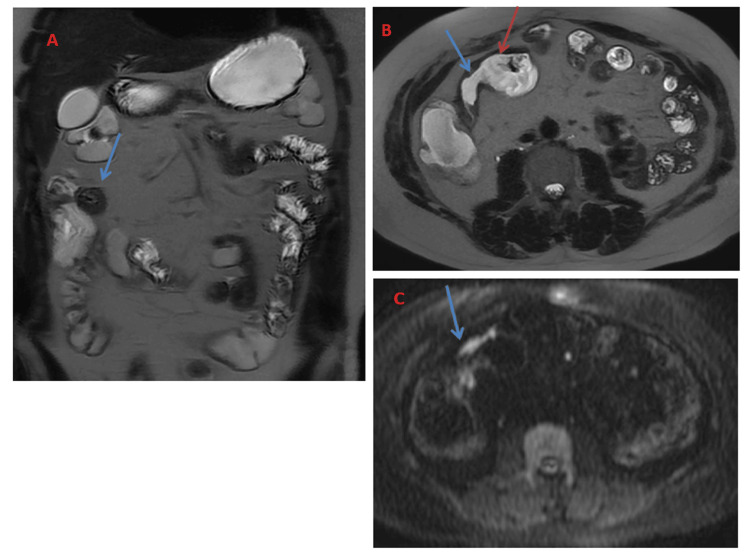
Magnetic resonance enterography showing terminal ileal wall thickening and stricturing disease. (A) Coronal T2-weighted MRI enterography showing asymmetric circumferential wall thickening of the terminal ileum. (B) Axial T2-weighted MRI enterography showing irregular stenosing wall thickening of the terminal ileum with digestive distension of the upstream loop. (C) Diffusion-weighted MRI enterography showing irregular wall thickening of the terminal ileum with hyperintense signal on diffusion.
